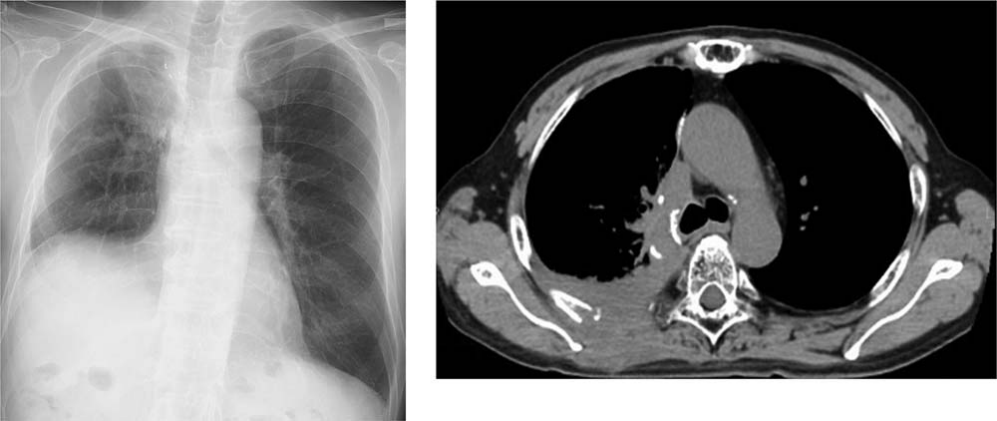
Chest X-ray and computed tomography (CT) at the initiation of treatment. CT scan reveals an osteolytic lesion and an abscess extending into the subcutaneous tissue.
